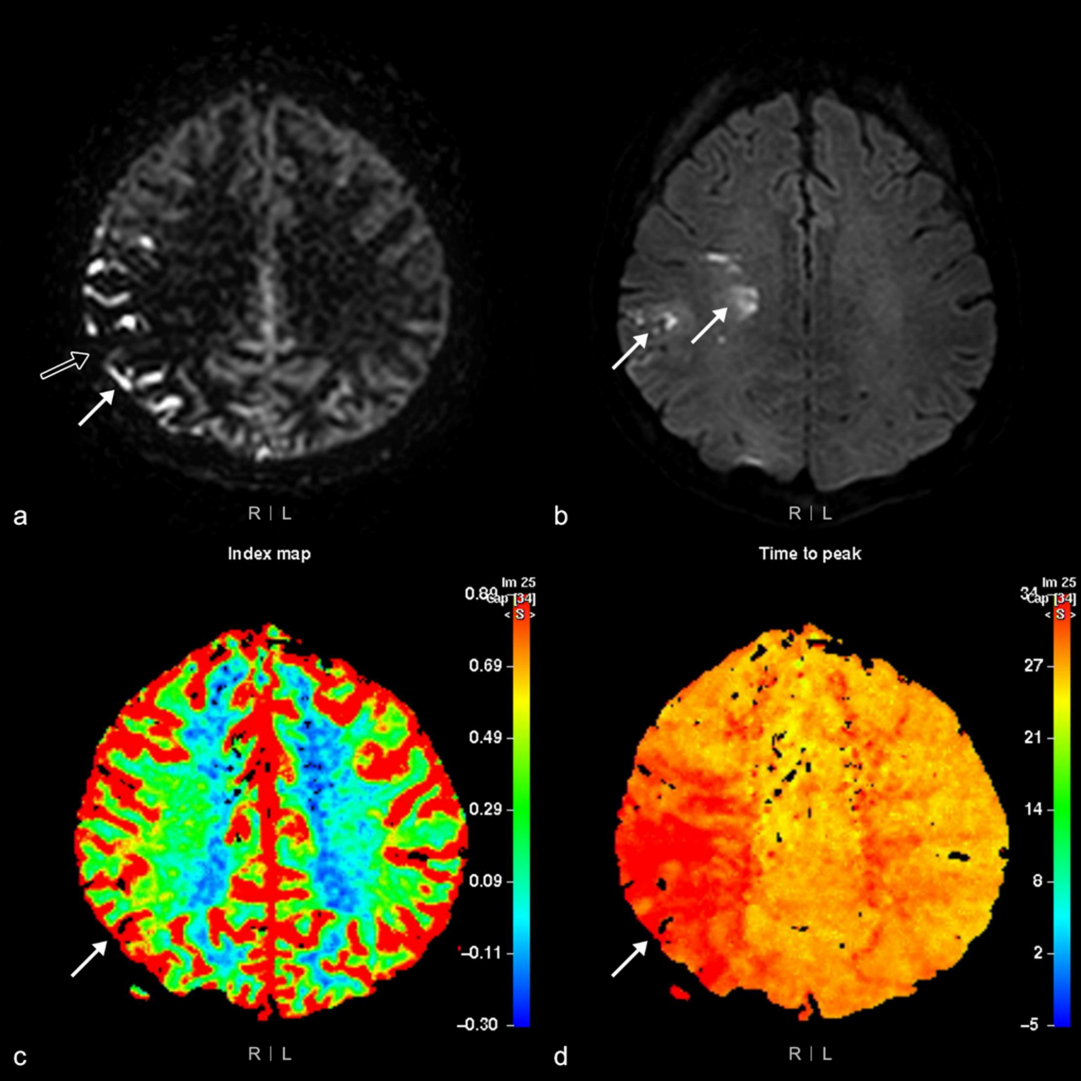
Arterial spin labeling (ASL) rank III perfusion abnormality. MRI of the brain of a 48-year-old woman with left-side motor weakness. The ASL image (a) shows a rank III perfusion abnormality, as hyperintense vessel signals (arrow) and parenchymal perfusion deficits (open arrow) are present in the right parietal area. However, high signal intensity (arrow) visualized by diffusion-weighted imaging (b = 1,000) (b) is detected only in the right frontal white matter and parietal area. Cerebral blood flow (c) and time to peak maps (d) show a mismatched perfusion defect (arrow) in the right parietal area.
